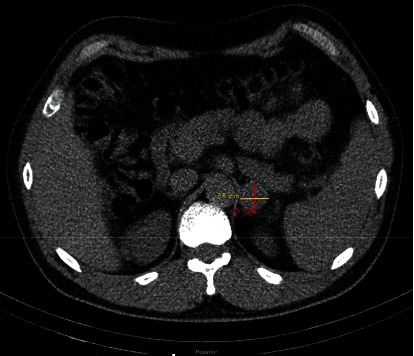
CT scan showing a 2.6 cm left adrenal mass.
